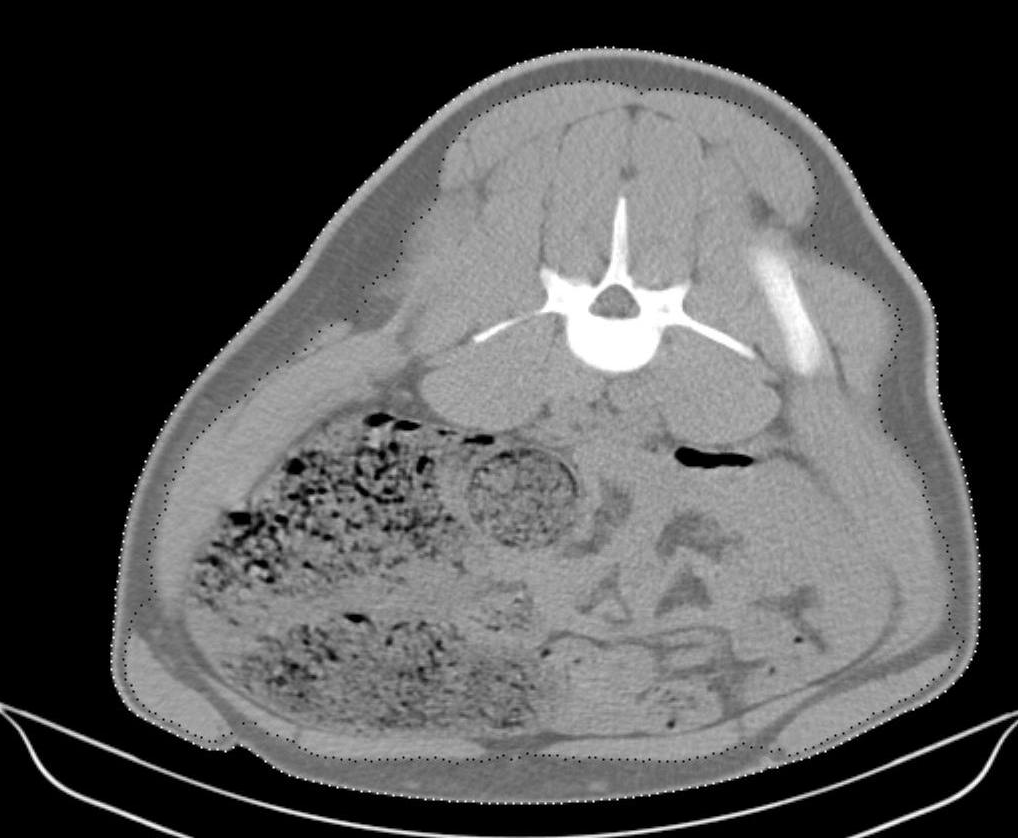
Computer tomography image. Transverse CT image showing the automatically determined boundaries. The outer limits of the skin are marked with white dots; the inner margin of subcutaneous adipose tissue is marked with black dots. Note in this particular image the algorithm misrepresents the desired boundaries in places because it was interrupted by discontinuities within the adipose tissue.
